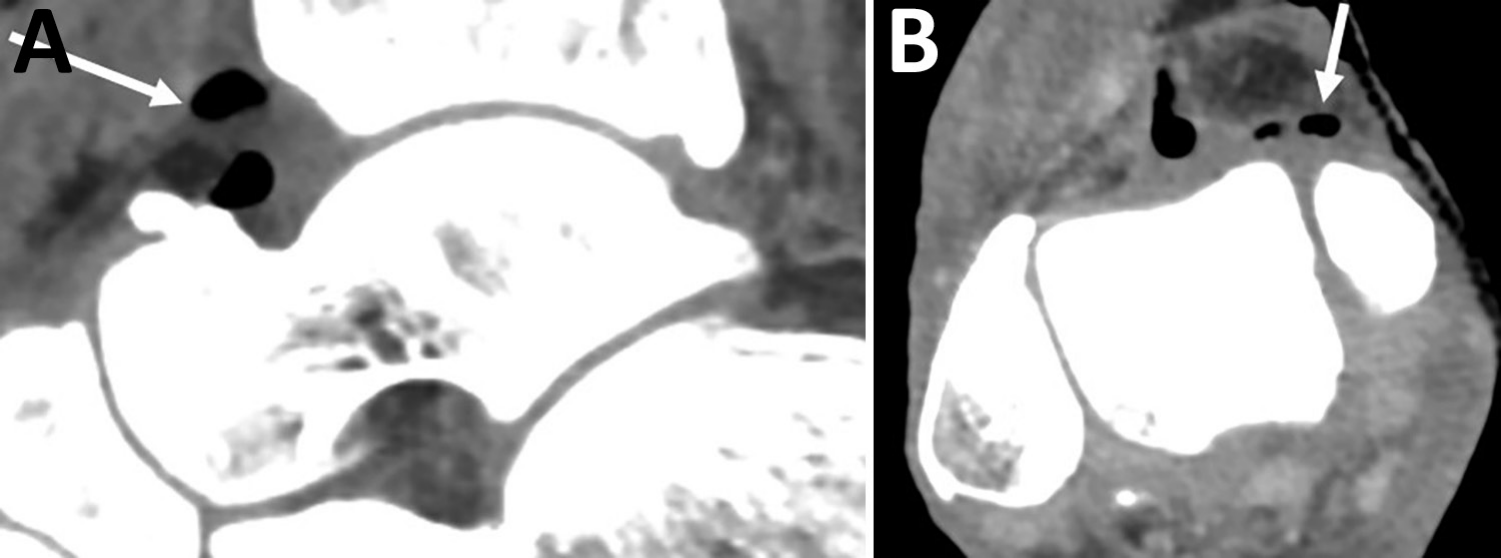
Sagittal (left) and axial (right) contrast-enhanced computed tomography images demonstrating ankle joint effusion associated with gas (arrows) in an immunocompromised man in France who had septic arthritis and soft tissue infection caused by *Nannizziopsis obscura.*
